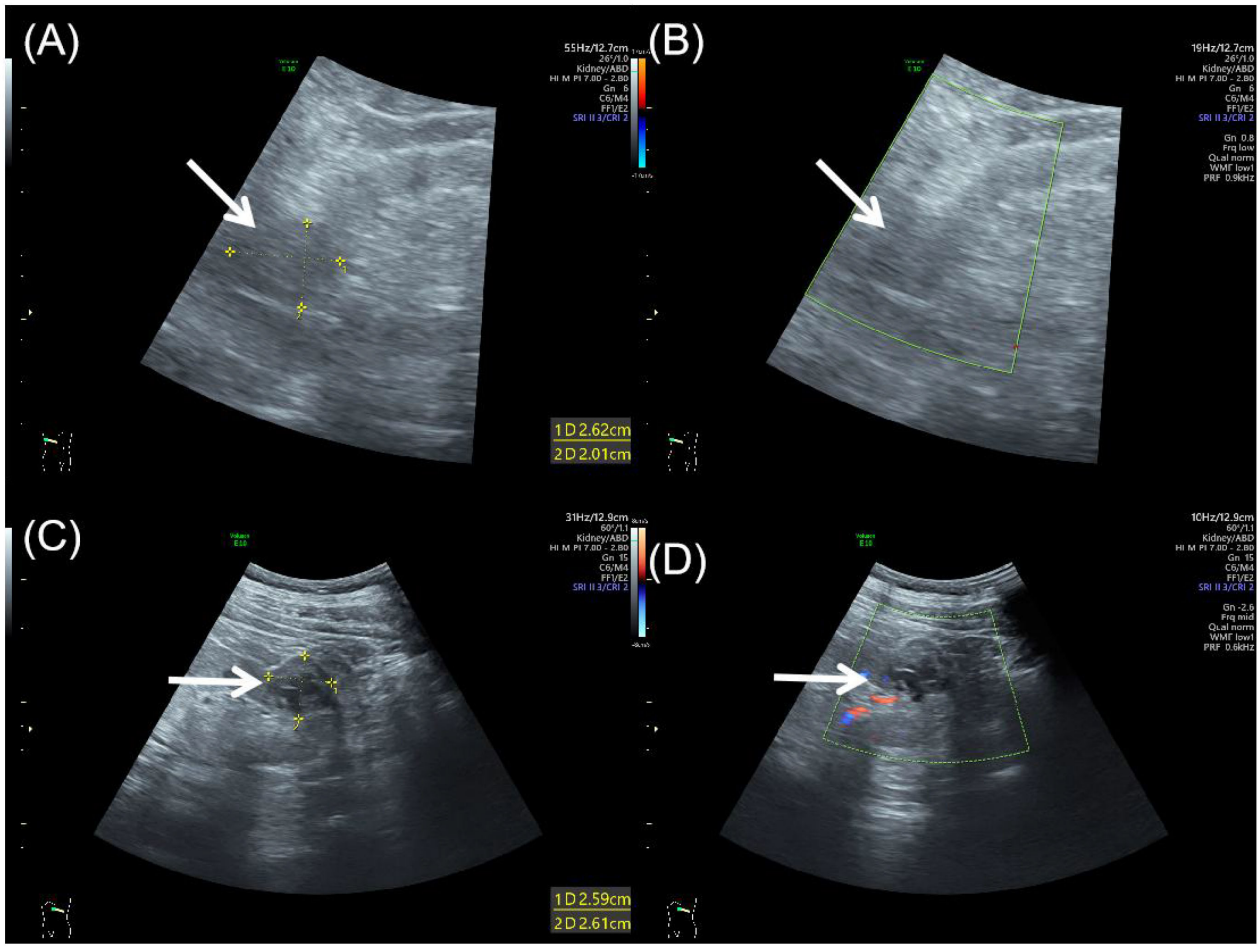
Abdominal ultrasound of bilateral synchronous rare renal tumors. (A) Grayscale ultrasound showed an oval, well-defined, heterogeneous mass (arrow) in the right kidney. (B) Color Doppler flow imaging showed no significant blood flow within the right renal mass (arrow). (C) Grayscale ultrasound showed a well-defined, heterogeneous cystic-solid mass (arrow) in the left kidney, with solid predominance and irregular hypoechoic areas. (D) Color Doppler flow imaging showed no significant vascularity in the left renal mass (arrow).
